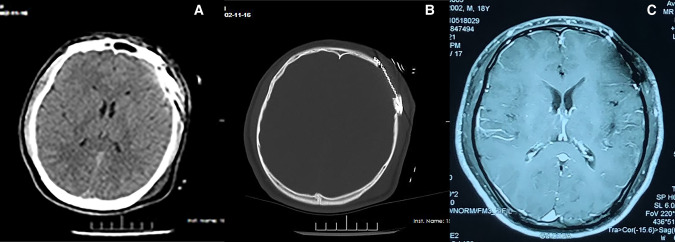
Imaging information were followed up one year after surgery of LCS (A,B) CT (C) MRI.
